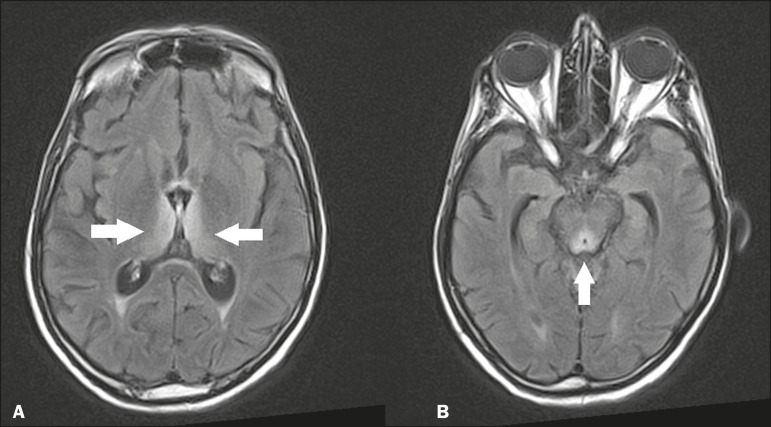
Wernicke’s encephalopathy in a malnourished alcoholic patient. A: Axial FLAIR MRI sequence showing symmetric areas of hyperintensity in the paraventricular regions of the thalamus (arrows). B: Axial FLAIR MRI sequence showing hyperintensity in the periaqueductal region (arrow).
